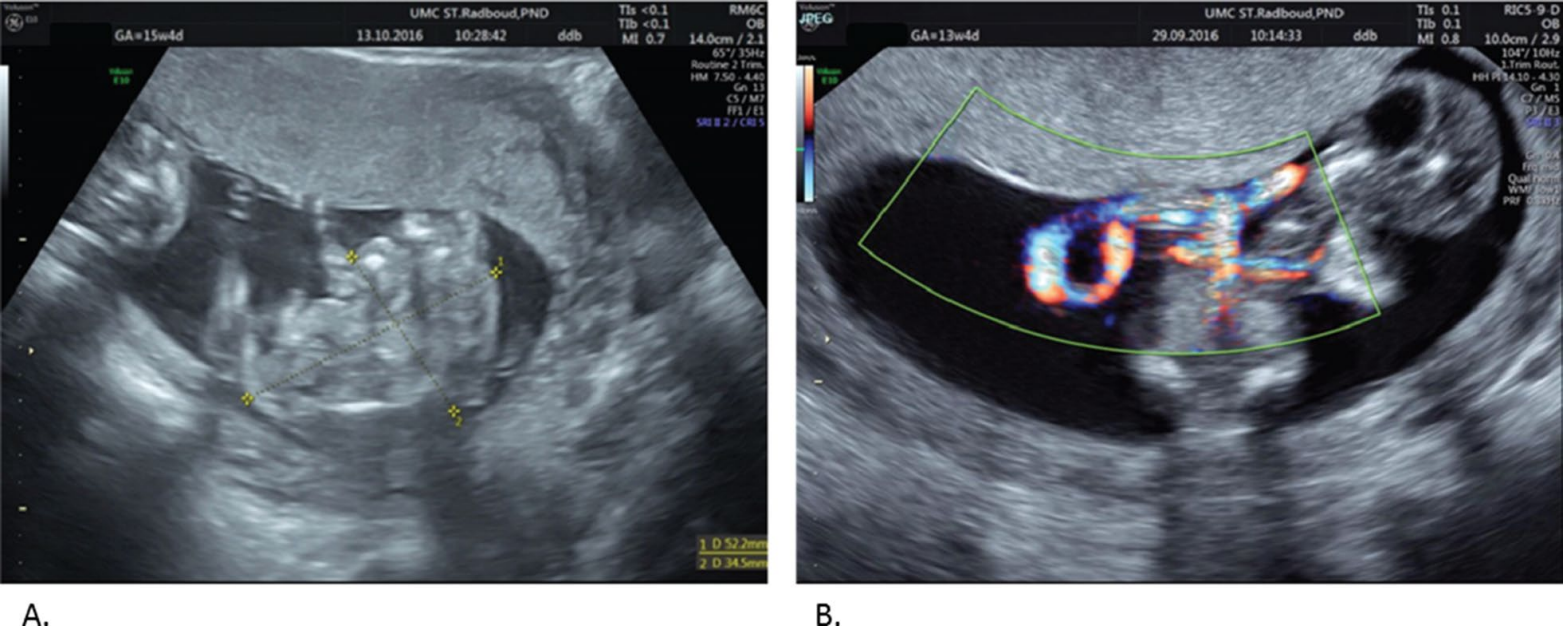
Ultrasonographic images of A acardiac twin and B cord entanglement, where blood flow is visualized
